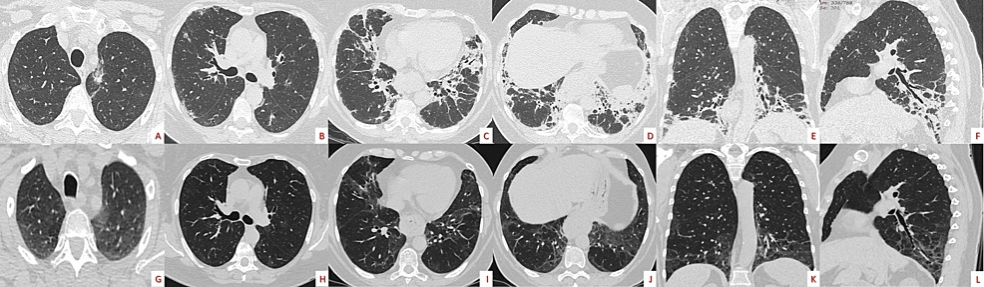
Serial follow-up high resolution computed tomographies Axial slices from lung apices to bases and coronal/sagittal images of chest computed tomographies show a radiological improvement with a decrease of bilateral ground glass opacities and patchy consolidations after one month of treatment; steroid naive (A-F), one month of treatment (G-L).
